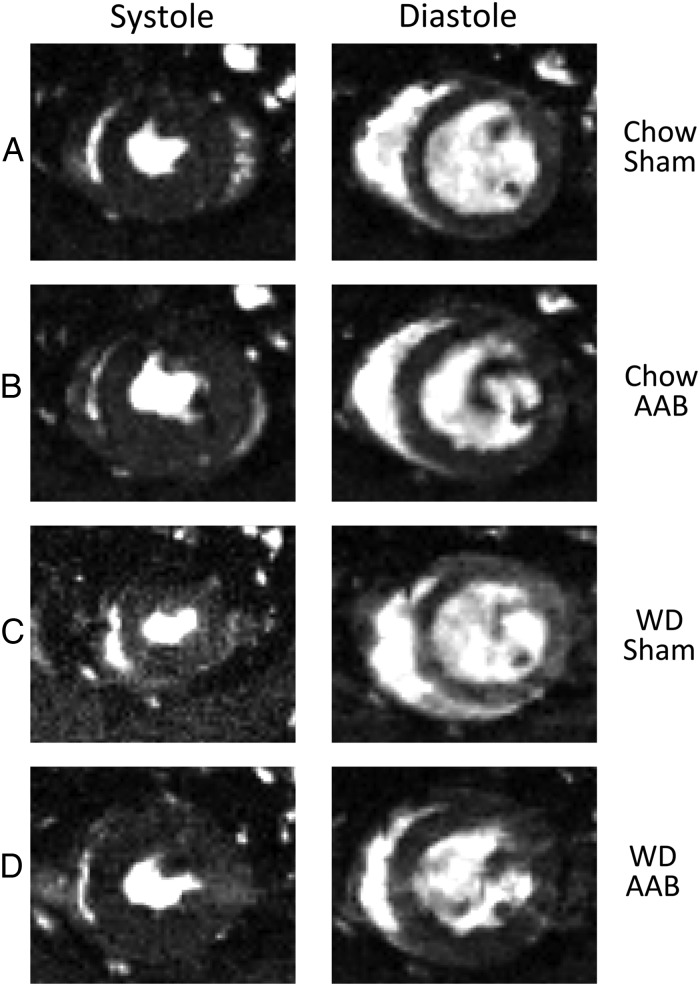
Representative *in vivo* CINE MR images of sham and AAB hearts at 14 weeks post-surgical induction of cardiac hypertrophy during systole and diastole, (*A* and *B*) on standard chow diet, (*C* and *D*) on WD.
